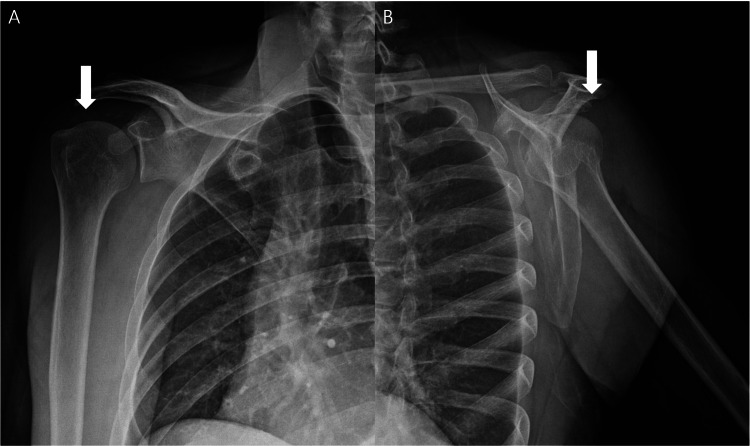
Near-complete radiographic resorption two weeks later. (A) Follow-up anteroposterior radiograph obtained two weeks after presentation shows near-complete resorption of the calcific deposit (arrow). (B) Follow-up scapular Y-view radiograph demonstrates marked reduction of the calcification (arrow).
